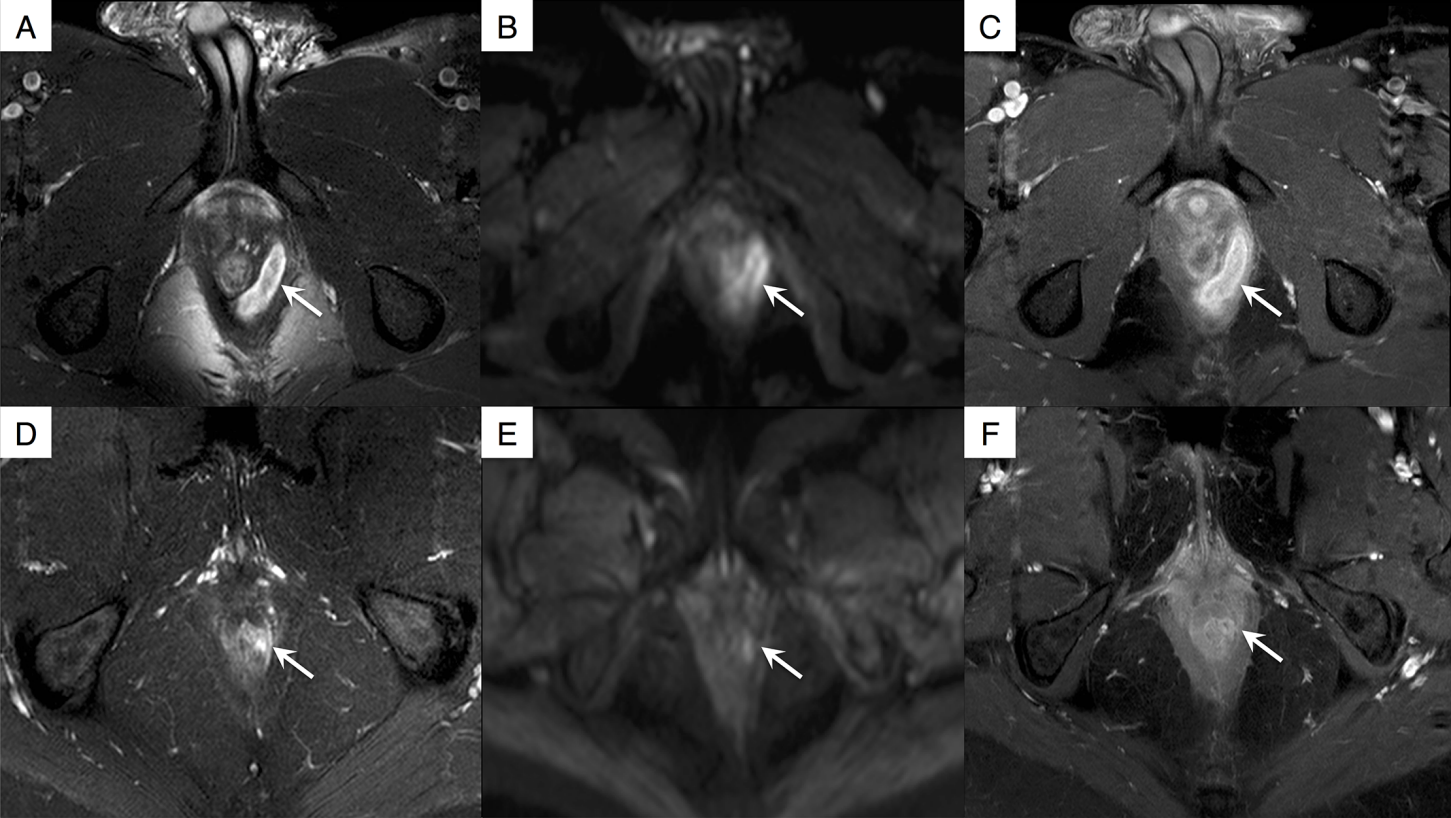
Representative images in 2 patients. Crohn's disease in a 21-year-old man with active fistula (arrows) shows (A) an inter-sphincteric abscess with marked hyperintense signal on the T2-weighted axial turbo spin echo sequence, (B) hyperintense signal on the IVIM-DWI sequence at *b* = 350 sec/mm^2^, and (C) marked enhancement on the contrast-enhanced sequence. Crohn's disease in a 46-year-old woman with inactive fistula (arrow) show (D) mild T2 hyperintense on the T2-weighted axial turbo spin echo sequence, (E) hypointense signal on the IVIM-DWI sequence at *b* = 350 sec/mm^2^, and (F) minimal enhancement on the contrast-enhanced sequence.
